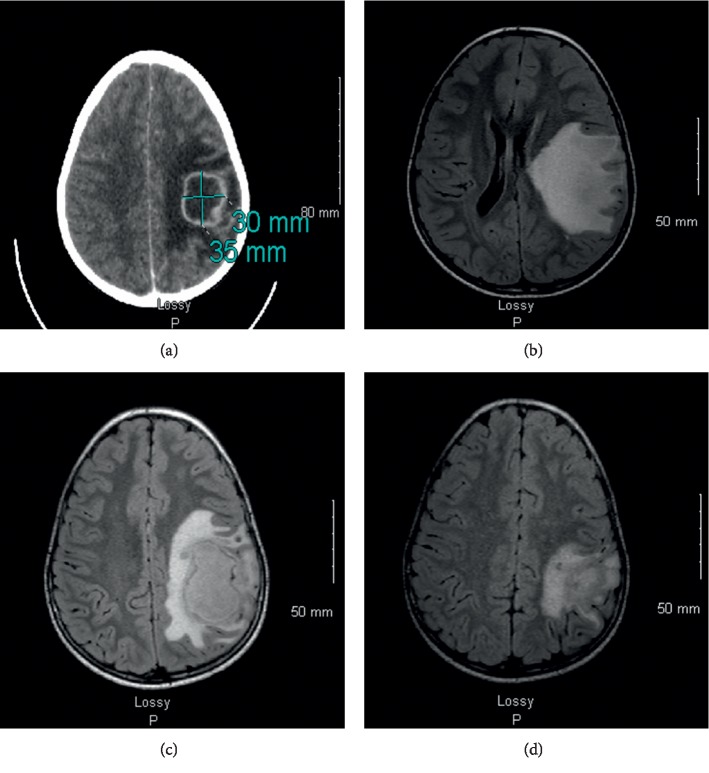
(a) Left fronto-parietal abscess revealed by a brain scan. (b) Axial view of the magnetic resonance imaging reveals a 45 × 52 mm abscess in the left front-temporal lobe of the brain (T0). (c) Axial view of the magnetic resonance imaging reveals an increase in the volume of the abscess, justifying a second drainage (*T* + 23 days). (d) Axial view of the magnetic resonance imaging shows a decrease in the abscess relative to the previous image (Figure 1(c)) (*T* + 2 months).
